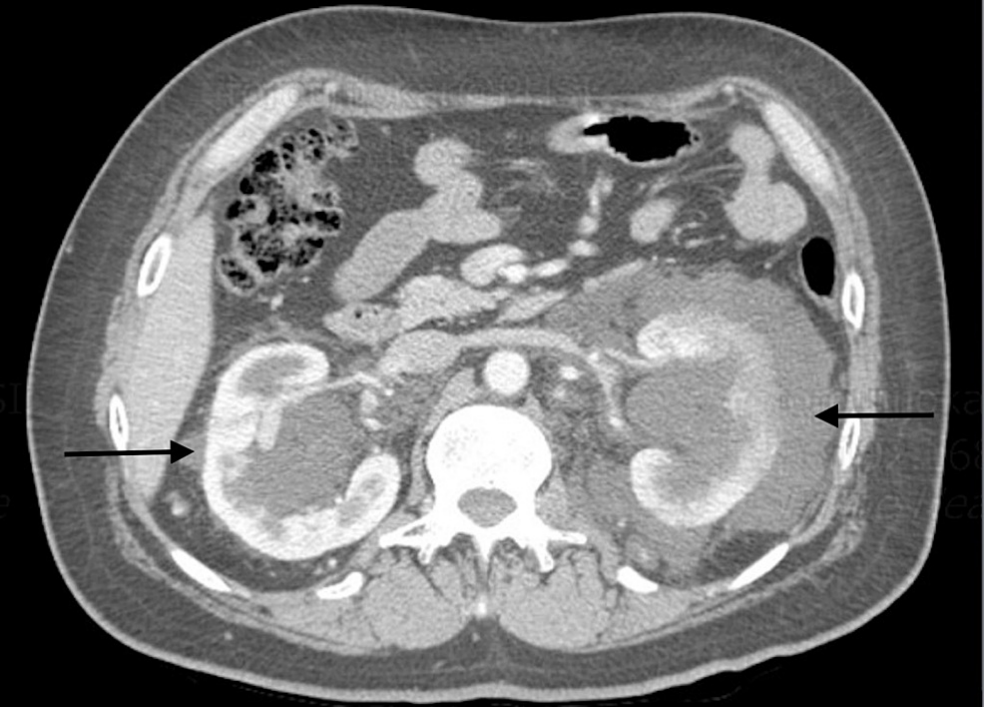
CT findings of lymphangiectasia: arrows pointing to perinephric lymphatic infiltration
